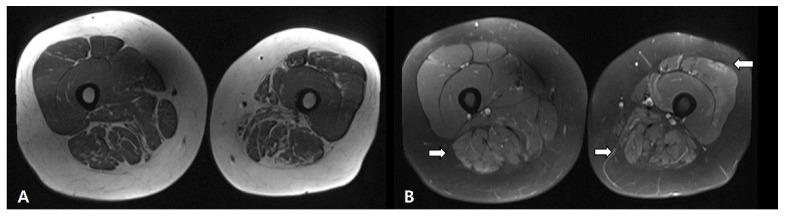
Results of magnetic resonance imaging (MRI) T1-dissusion (A) and T1-fat suppression (B) images of both thighs demonstrated diffuse high signal intensities on both thigh muscles (white arrows) and atrophy in the left buttock and thigh muscles.
